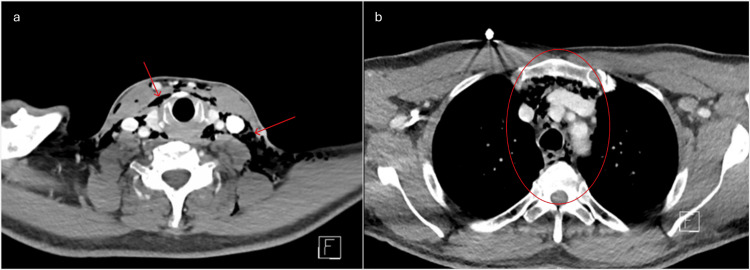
Multiple CT scan views of the thoracic area: (a) emphysema surrounding the neck vessels and the trachea and (b) emphysema extending into the mediastinum CT: computed tomography
